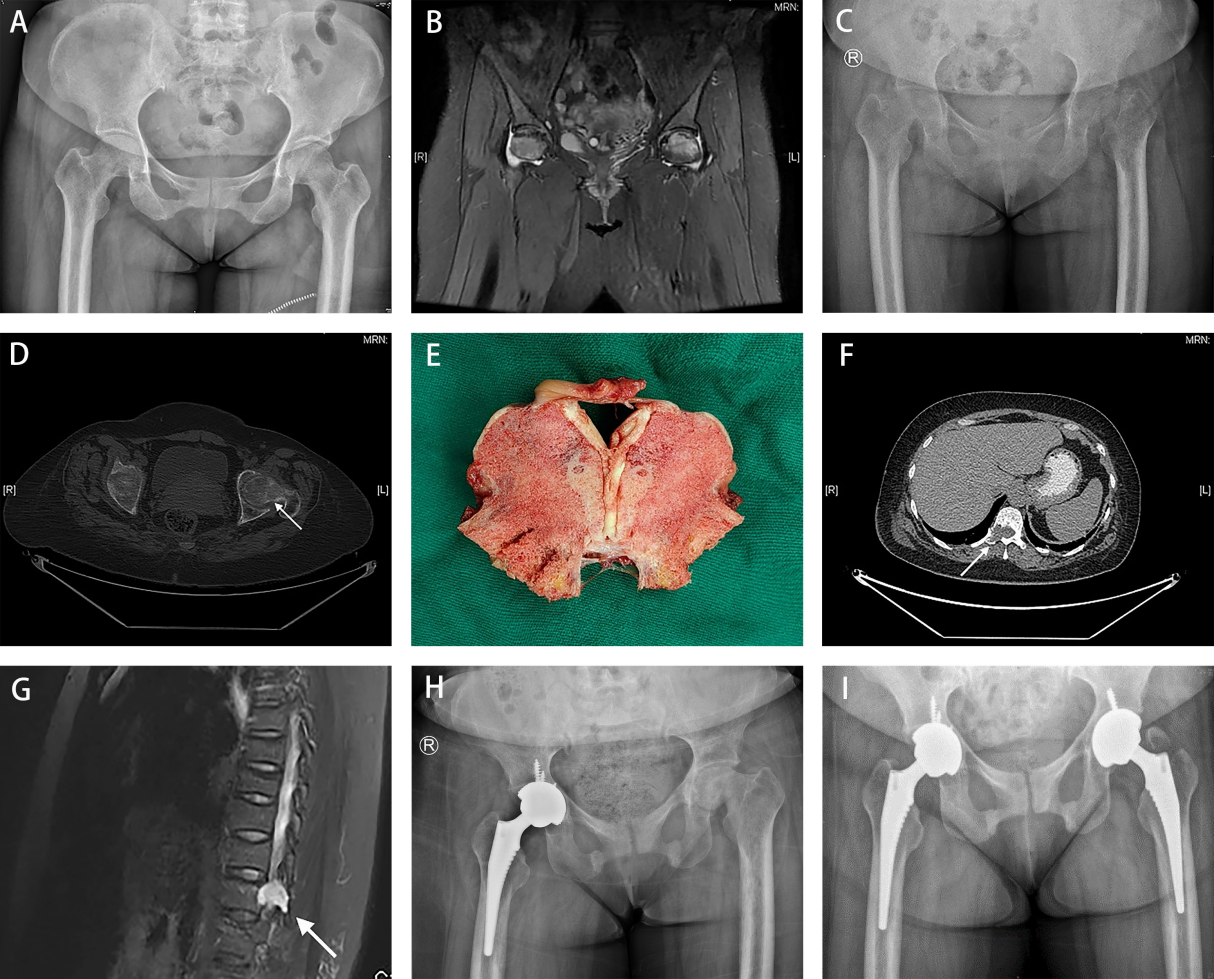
A 49-year-old woman presented consistent bone pain with limb weakness for more than 2 years. (A) The pelvic AP radiograph presented grossly normal hips in June 2020. (B) Magnetic resonance imaging revealed low-signal intensity bands in the bilateral femoral head. (C, D) Images presented blurred bilateral hips with insufficiency femoral neck fracture in June 2022 (indicated by white arrow). (E) Severe osteomalacia was found in the femoral head sample. (F, G) A well-circumscribed lesion of about 13×18-mm in size was observed in the T11 by PET/CT( white arrow in F) and MRI with long T1 and T2 signals (white arrow in G) in August 2022. (H) Radiographs obtained in September 2022 demonstrated the narrow space and coxa vara of the left hip, and proper prosthetic placement without loosening in the right hip. (I) Radiographs obtained in March 2023 showed proper bilateral prosthetic positions without signs of loosening.
